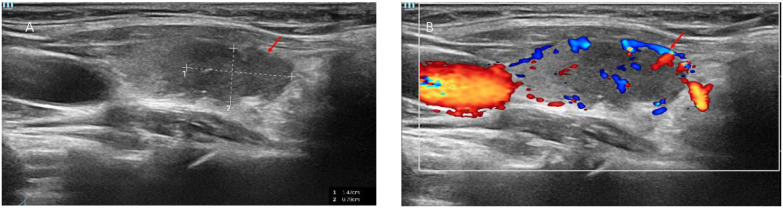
Thyroid ultrasound of the thyroid metastasis. (A) Gray-scale ultrasound shows a hypoechoic lesion in the right lobe, measuring 14.2 × 7.9 mm (calipers, lower right corner). (B) Color Doppler image demonstrates internal vascularity within the lesion.
